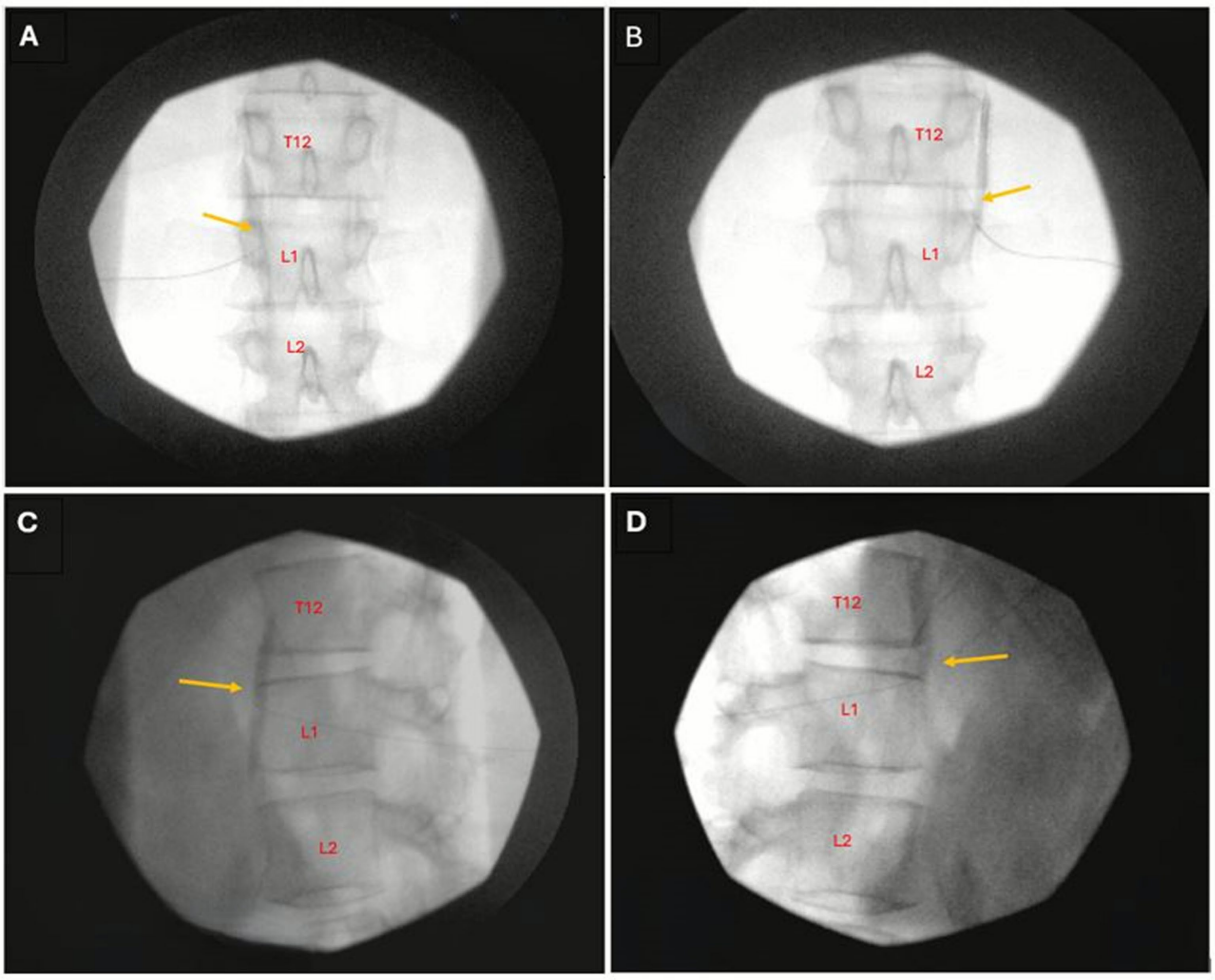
Radiographs demonstrating the posterior retrocrural approach for celiac plexus block. (A,C) Left-sided approach. (B,D) Right-sided approach. (A,B) Anteroposterior view. (C,D) Lateral view. T, thoracic vertebra; L, lumbar vertebra. Yellow arrows indicate contrast spread at injection site.
